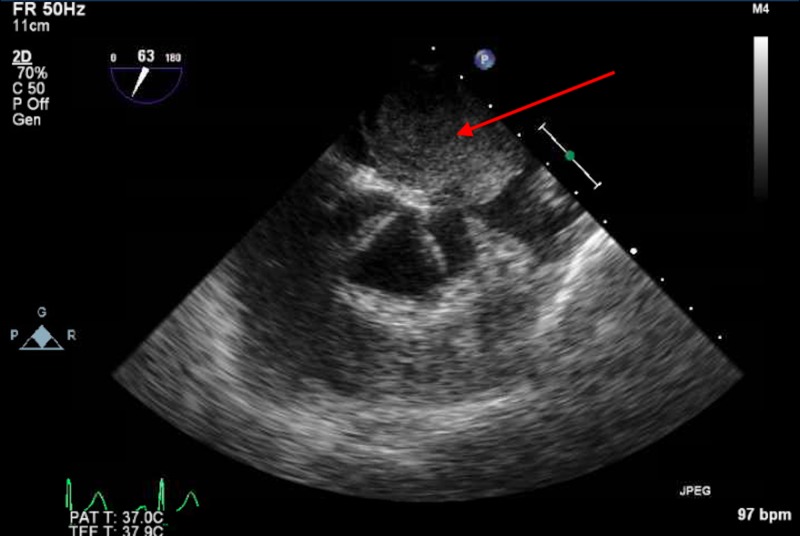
Transesophageal echocardiographic image showing left atrial mass (red arrow) seated above the aortic valve
